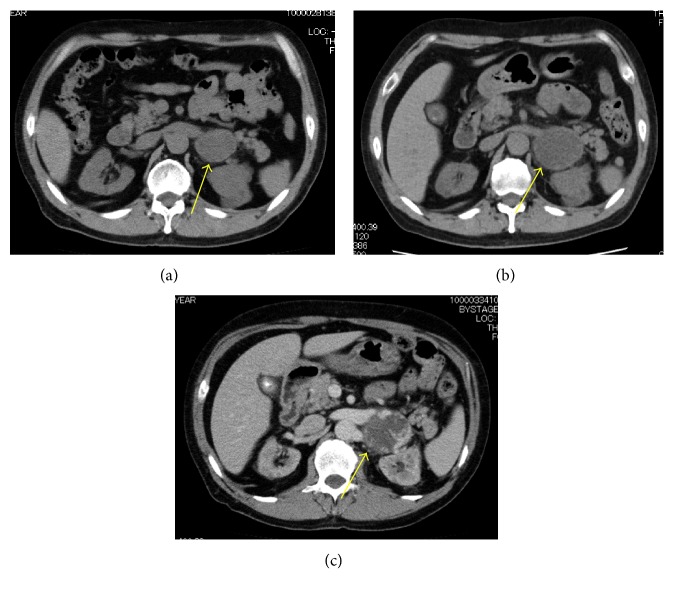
CT images. The tumor developed gradually.
